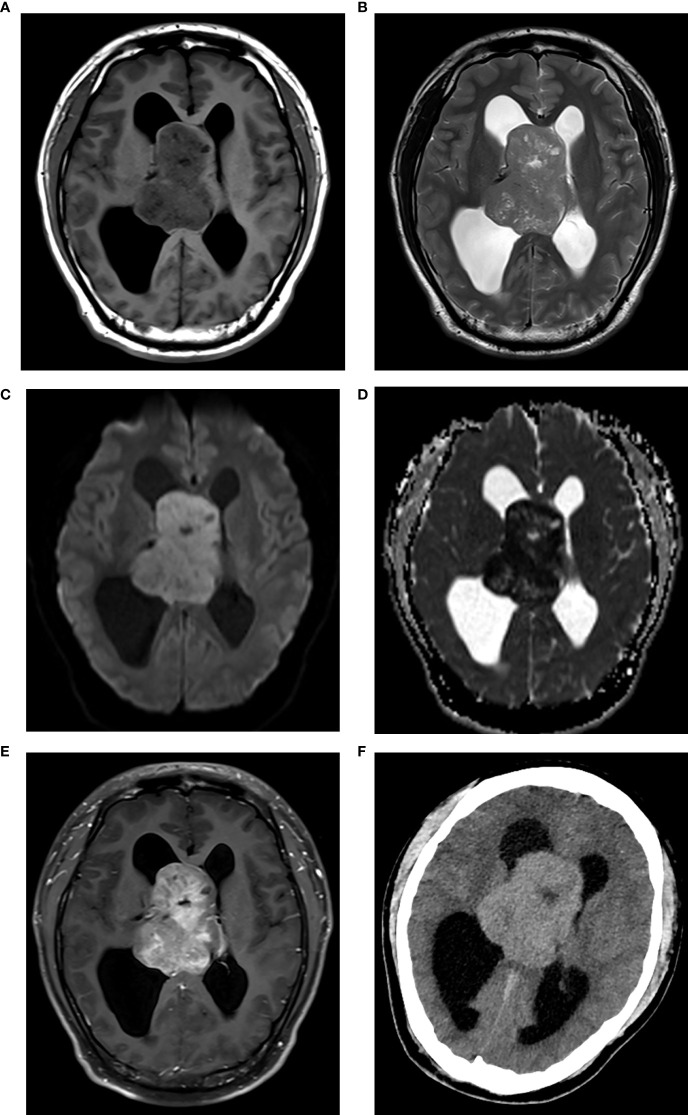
CN with high Ki-67. Axial MRI scans revealed a solid lesion around the septum pellucida, showing isointensity on T1WI (A) isointensity on T2WI (B) hyperintensity on DWI (C) hypointensity on ADC map [(D) the mean ADC = 0.73×10–^3^ mm^2^/s], and marked enhancement on contrast-enhanced T1WI (E). The lesion protruded into both lateral ventricles and caused hydrocephalus. The lesion showed hyperdensity on axial CT [(F), CT density = 46 Hu].
